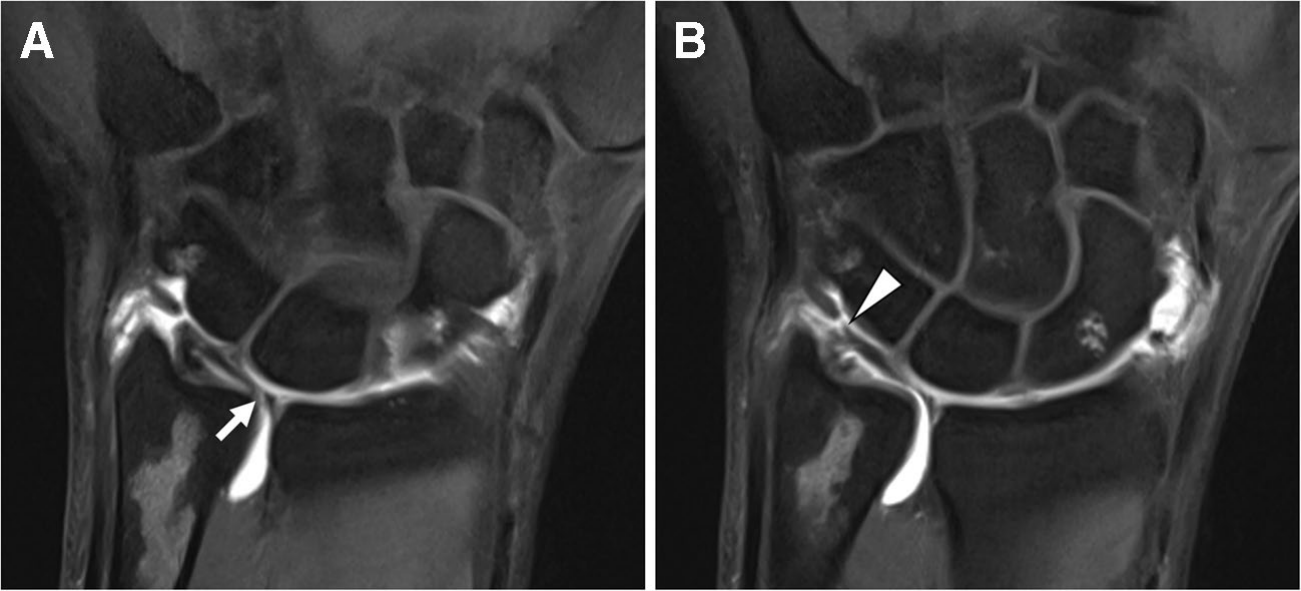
Triangular fibrocartilage complex (TFCC) injury. A, B Coronal T1-weighted 3T MR arthrogram images show communication between the radiocarpal and distal radioulnar joints through a tear of the TFCC involving both the central disc (arrow) and ulnar attachments (arrowhead). Findings were confirmed at arthroscopy and an open foveal repair was performed
